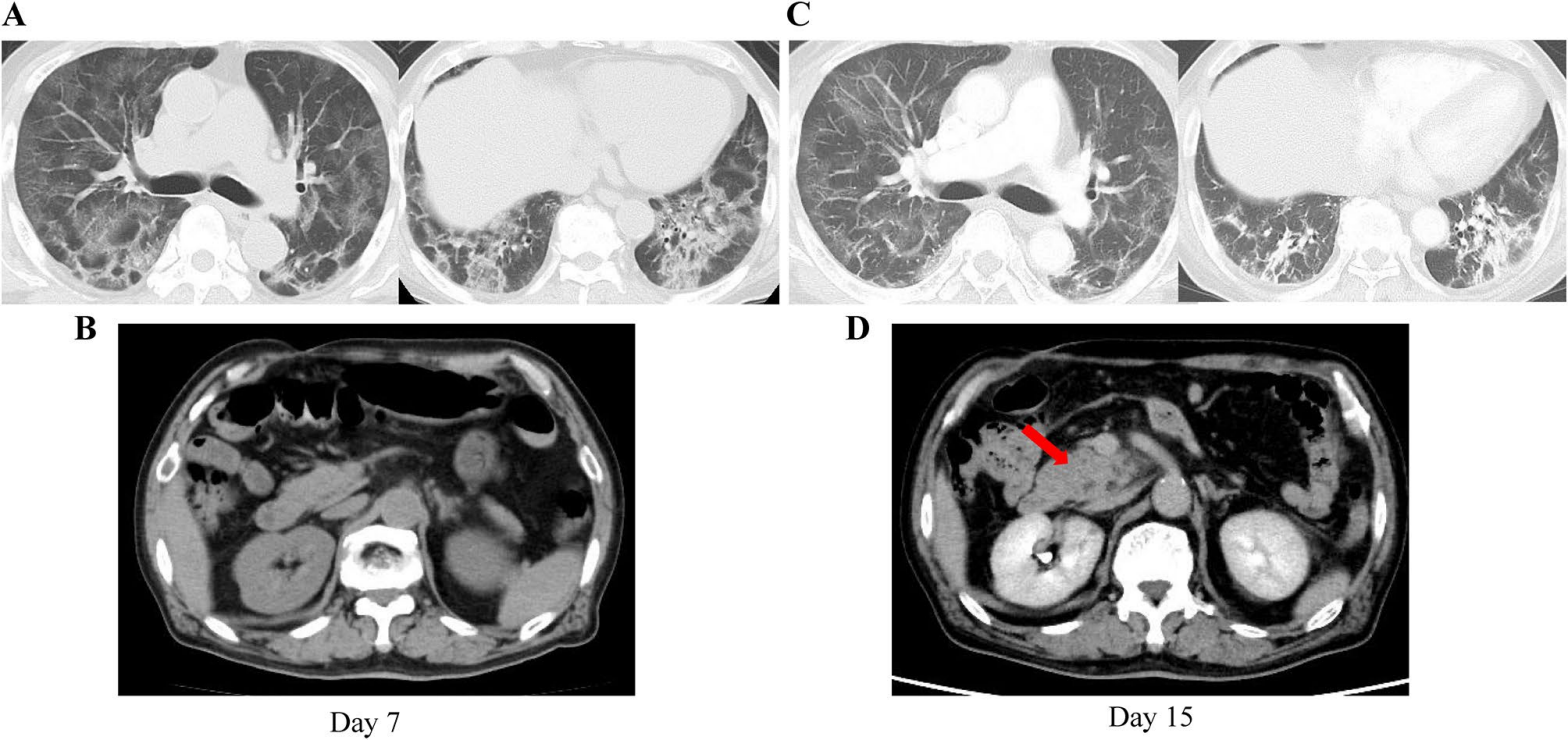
Computed tomography (CT) scan of a patient who developed acute pancreatitis after remdesivir administration. This is one of the three cases of acute pancreatitis. CT was performed at the time of admission to our hospital (7 days after COVID-19 onset) and 15 days after COVID-19 onset, when an increase in pancreatic enzyme levels was observed. (A) During hospitalization, extensive ground-glass shadows were observed in the bilateral lung fields. (B) Abdominal CT on day 7. (C) However, the ground-glass shadows dissipated slightly by day 15, showing some contractile changes. (D) Contrast-enhanced CT image was obtained when pancreatic enzyme levels were elevated, and it shows an enlarged uncinate process of the pancreas and an increase in the concentration of surrounding fatty tissue, which were not observed at the time of admission (B).
